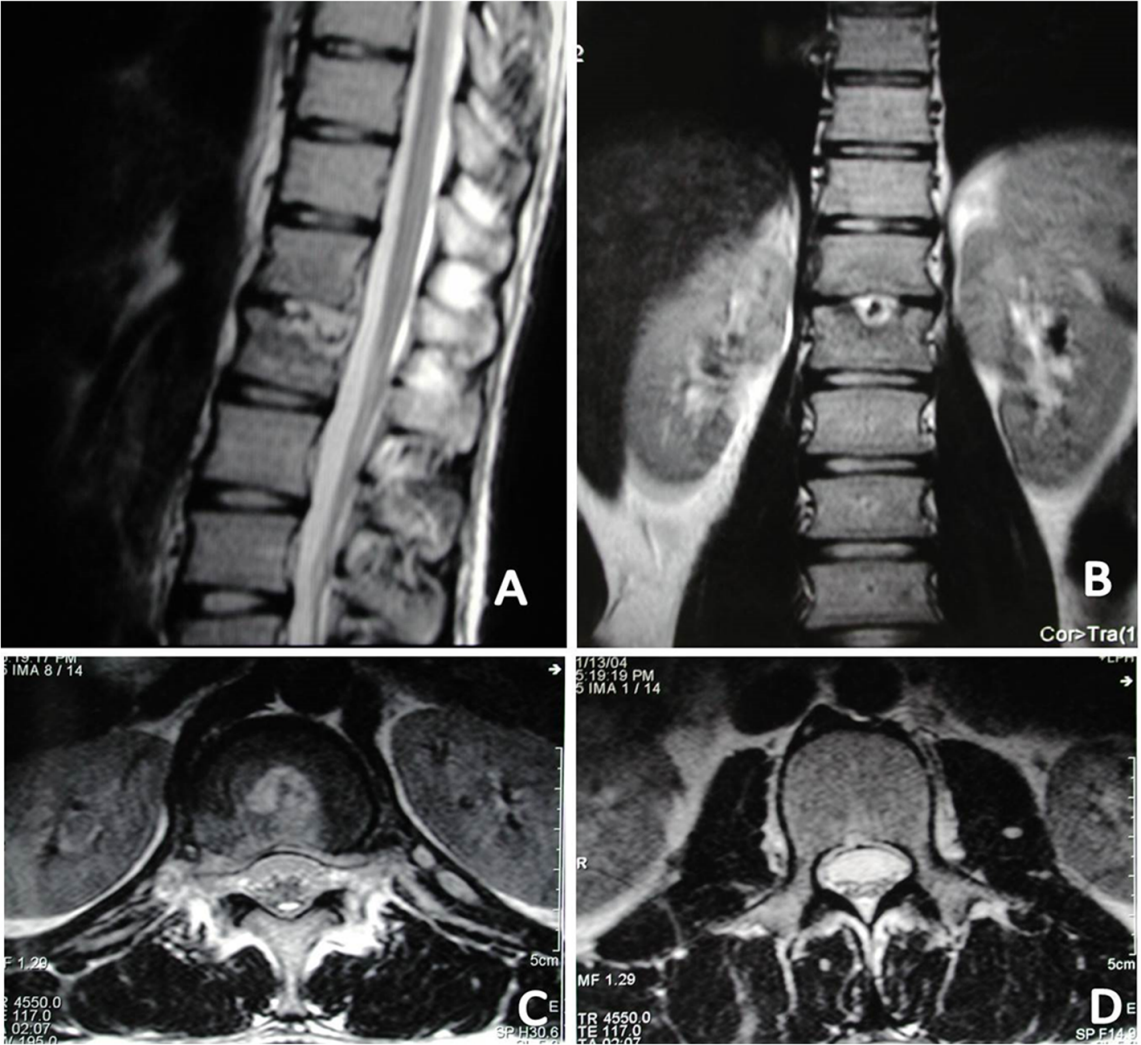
24 months after PCD and PCI chemotherapy, The MRI showed that intervertebral lesion were totally under control (a) with the right kidney was back to its right place and no abscess were observed in the bilateral psoas muscles in the coronal MRI (b) and in the axial MRI (d), and that the para-vertebral abscess also disappeared and the spinal cord were decompressed
